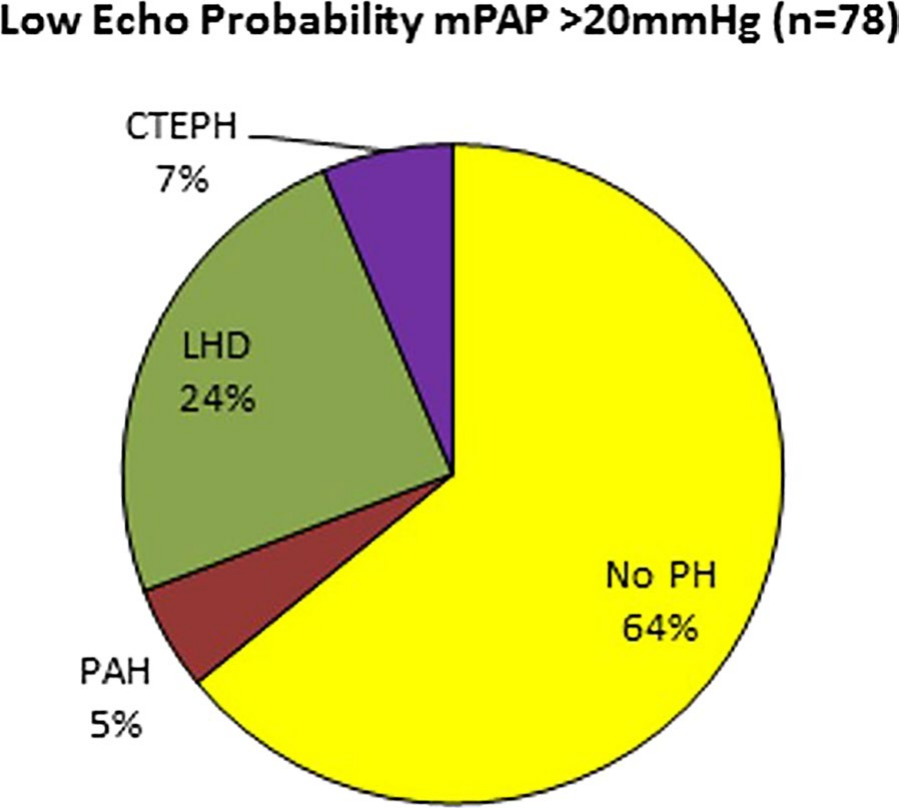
Comparison of the frequency of WHO Classification of PH in those with an low echocardiographic probability of PH (N = 78)
